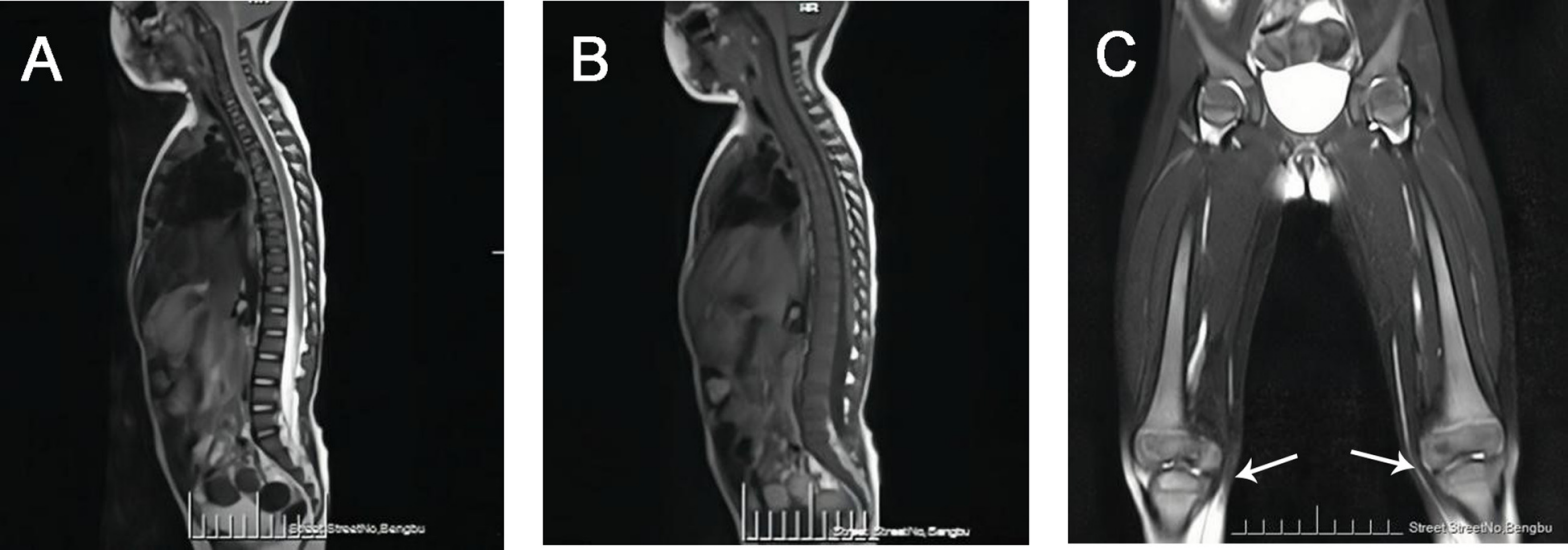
(A) MRI-T2WI image demonstrated diffusely decreased signal intensity in the vertebral bodies. (B) MRI-T1WI image demonstrated diffusely decreased signal intensity in the vertebral bodies. (C) Bilateral femoral MRI demonstrated metaphyseal widening of the distal femora with flask-shaped morphological alterations (white arrow).
